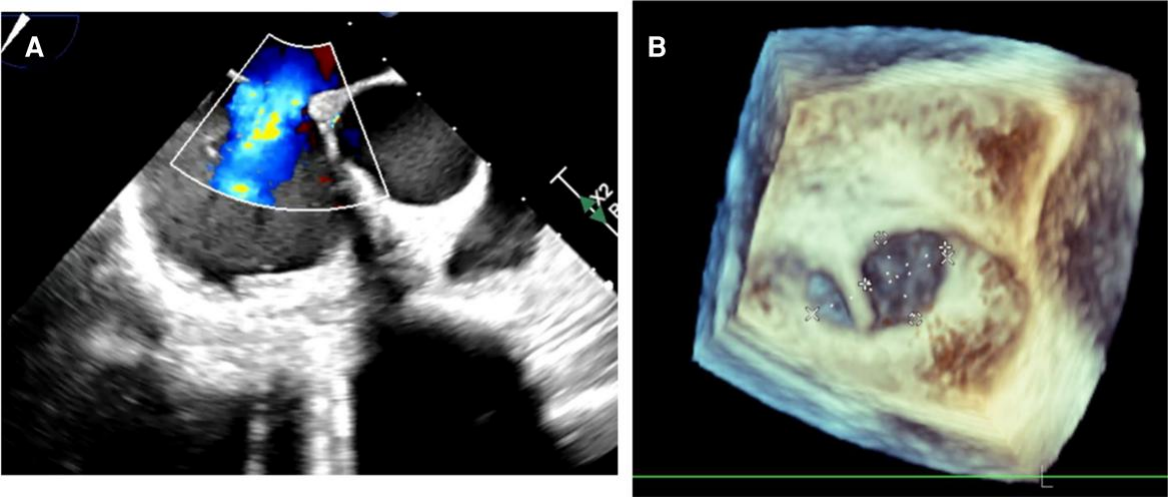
Transoesophageal echocardiogram showing (*A*) two-dimensional image of secundum atrial septal defect with left-to-right shunt and (*B*) three-dimensional reconstruction of atrial septal defect measuring 16 × 8 mm with muscular band separating two sides.
